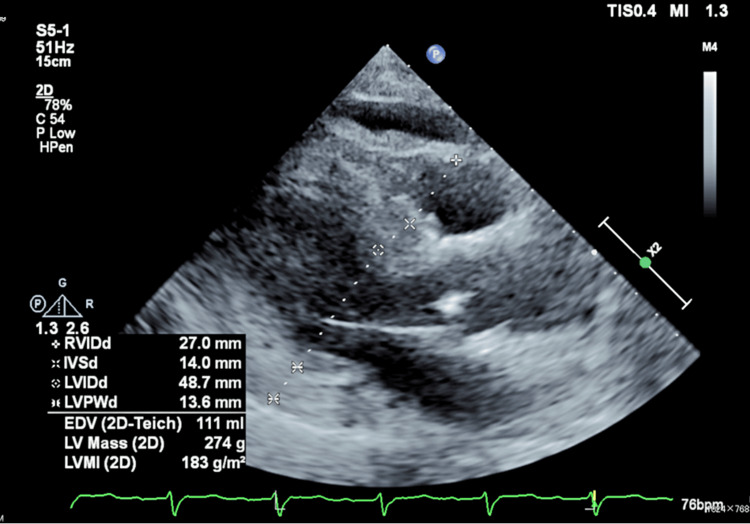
Findings of transthoracic echocardiography Left ventricular hypertrophy can be seen with interventricular septal end diastole (IVSd) of 14.0 mm and left ventricular posterior wall end diastole (LVPWd) of 13.6 mm. Right ventricular internal dimension diastole (RVIDd) was 27.0 mm. Left ventricular internal dimension diastole (LVIDd) was 48.7 mm. End-diastolic volume (EDV) was 111 mL. Left ventricular (LV) mass was 274 g. LV mass index (LVMI) was 183 g/m^2^.
